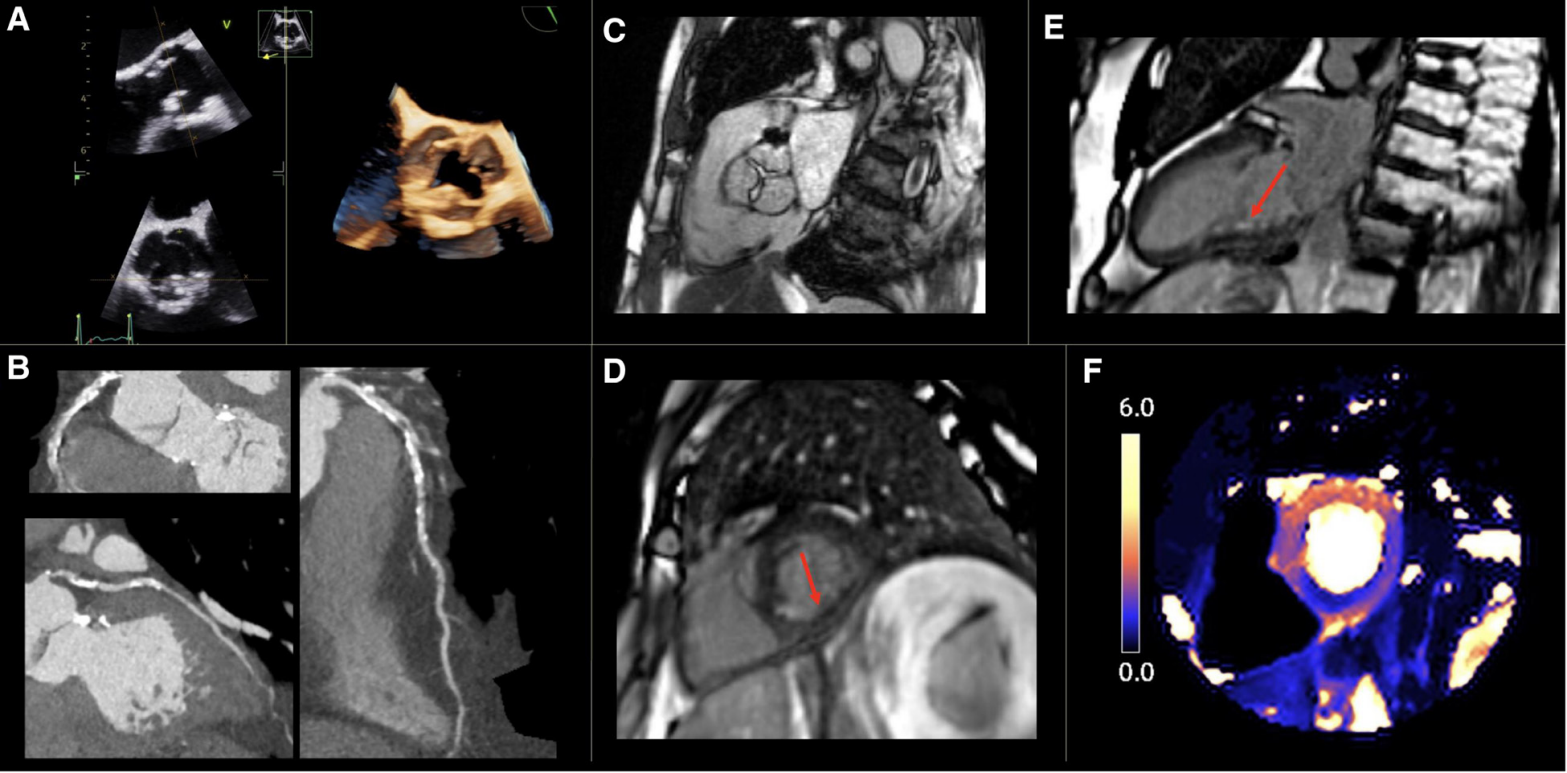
Multimodality imaging demonstrating radiation-induced cardiovascular toxicity in a 72-year-old man who had mantle radiotherapy aged 38 years for Hodgkin lymphoma. Patient had presented with exertional breathlessness and chest pain with transthoracic echocardiogram demonstrating aortic stenosis. Transesophageal echocardiography (A) demonstrated typical radiotherapy-induced changes of the aortic valve, while computed tomography coronary angiogram (B) showed diffuse coronary disease with severe disease in proximal left anterior decending, left circumflex and right coronary arteries. Patient went on to have cardiovascular magnetic resonance imaging which showed severe aortic stenosis through planimetry of the valve on cine imaging (C), late gadolinium enhancement in the basal-mid inferior walls (arrows) suggesting subendocardial infarction (E and D), and adenosine-induced reductions in stress myocardial blood flow in the basal inferior, inferolateral, and inferoseptal segments using quantitative perfusion mapping (F).
